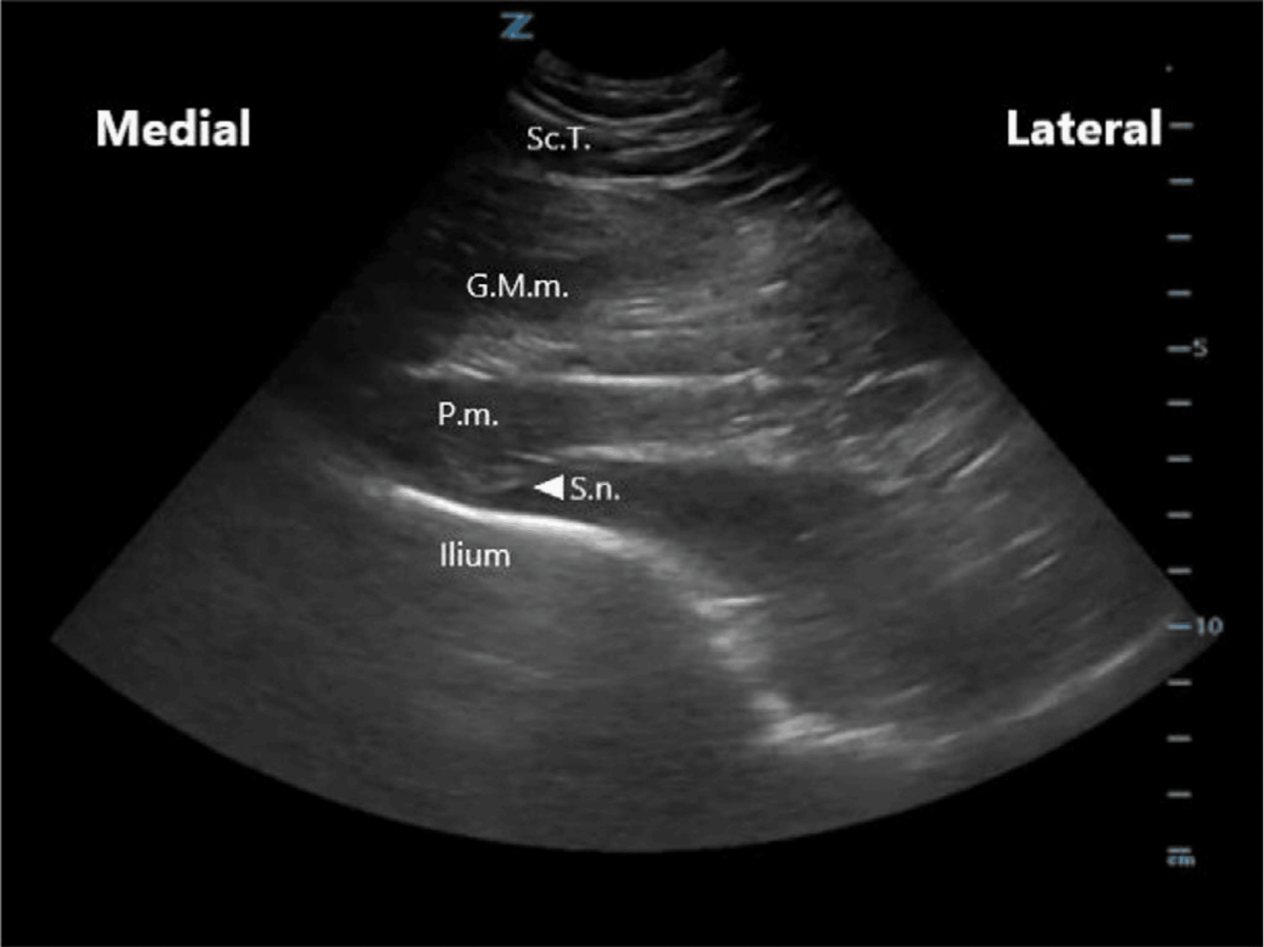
Anatomy of the piriformis muscle on ultrasound. Sc.T: subcutaneous tissue; G.M.m: gluteus maximus muscle; P.m: piriformis muscle; S.n: sciatic nerve [9].
